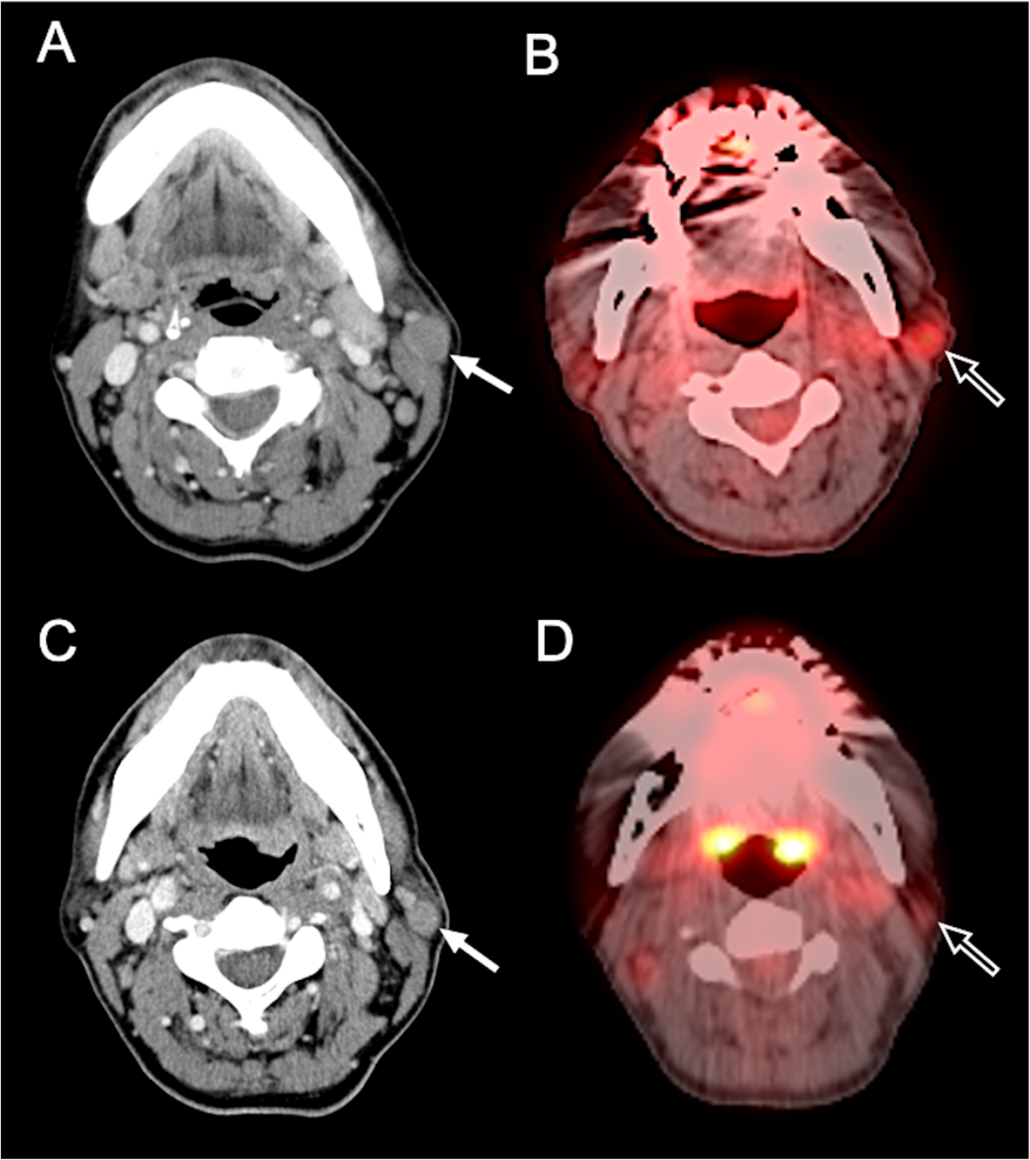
^18^ F-fluorodeoxyglucose (FDG) positron emission tomography (PET) can predict early response in targeted therapy. A 53-year-old man was diagnosed with Hodgkin lymphoma at the left parotid gland. A Pretreatment CE axial CT imaging shows a 16 mm tumor mass at the left parotid gland (white arrow). B The SUVmax value of 3.0 in the target lesion (open arrow) was detected on a pretreatment PET-CT scan. C After five cycles of brentuximab vedotin, a tumor size of 13.5 mm (white arrow) was observed, showing a 16 % reduction in size. According to the RECIST 1.1, this is a non-responder. D However, posttreatment PET-CT scan shows good tumor response with 50 % reduction of SUVmax value (from 3.0 to 1.5) (open arrow)
